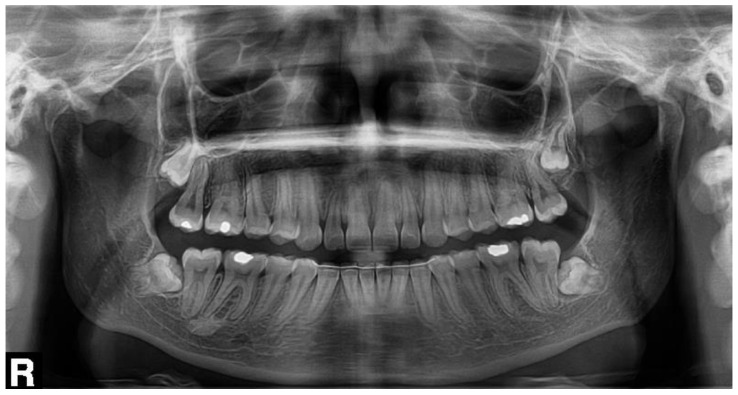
The orthopantomogram of the patient 1. Tooth No. 38 is semi-impacted in a mesially inclined, almost horizontal position. There is a burnout around the crown of the tooth.
